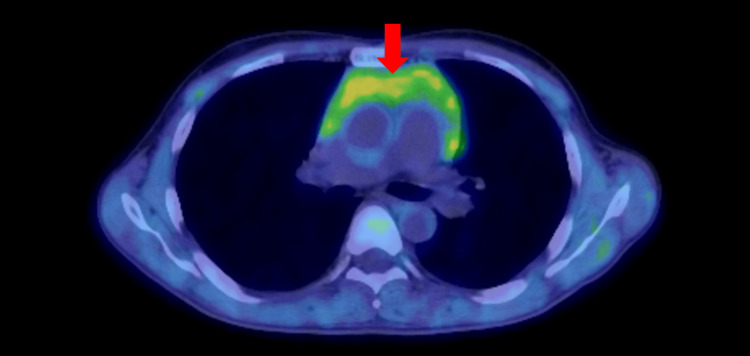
Positron emission tomography-computed tomography in the patient
